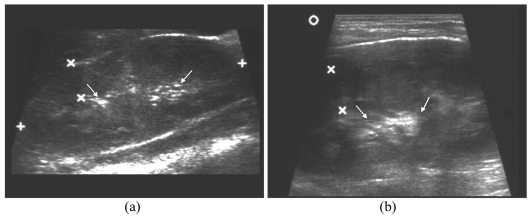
A six year-old girl had history of three urinary tract infections. a) Right renal sonogram shows compact sinus echoes (arrows); b) ECS shows no evidence of microbubbles and no PCS dilatation (arrows). The MCU showed right Grade II VUR.
